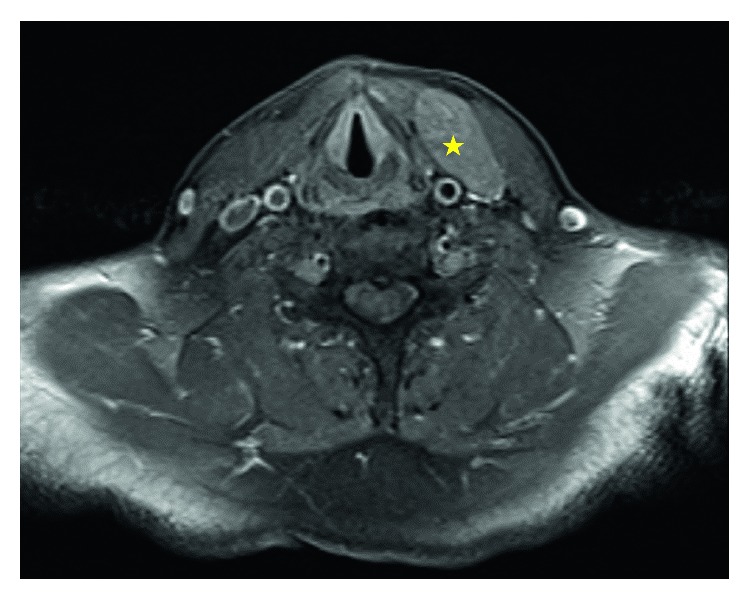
MRI T1 FatSat axial showing lesion (star) within left omohyoid muscle.
